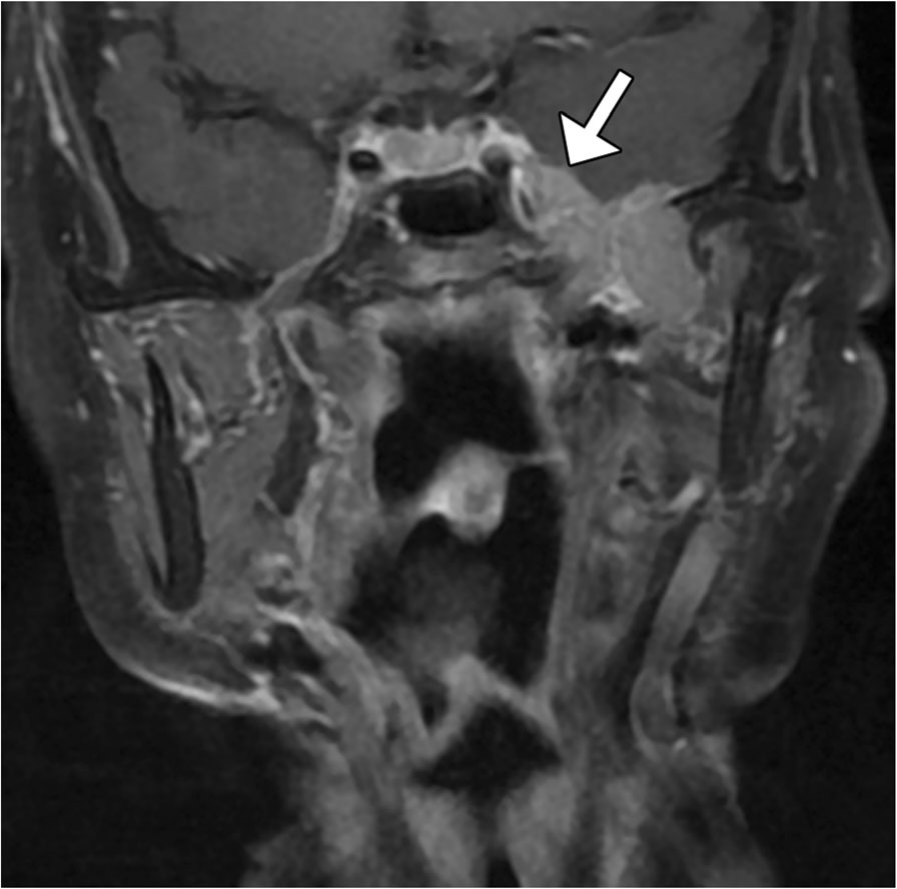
High-grade transformation of polymorphous low-grade adenocarcinoma originating from the parotid gland with perineural spread. Coronal fat-suppressed post-contrast T1-weighted MRI shows a mass in the left intratemporal fossa that extends through a widened foramen ovale into the left cavernous sinus (arrow)
